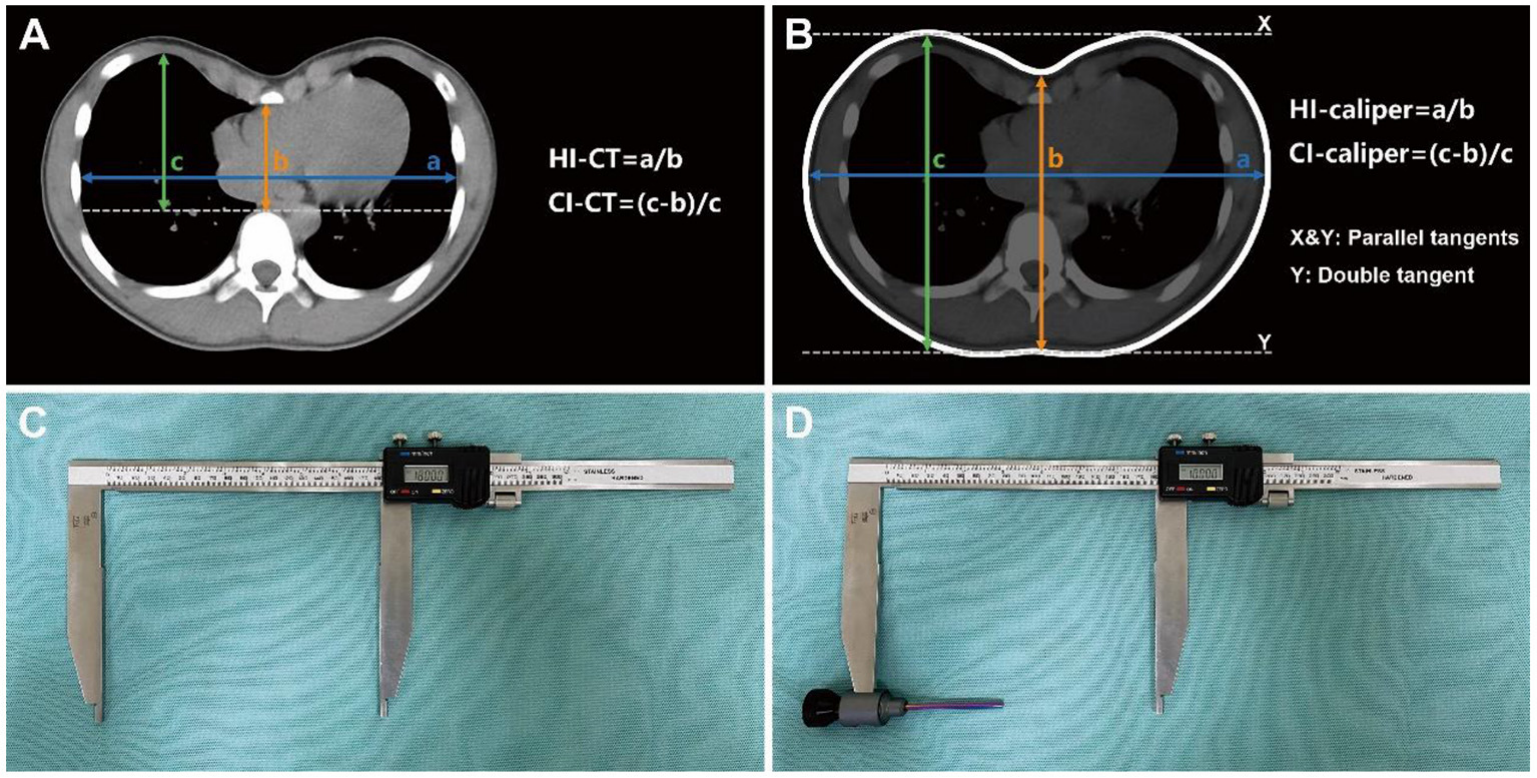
Severity indices and measuring caliper. (A) CT-based severity indices of internal measurement, targeting the bony thorax from pleura to pleura. (B) Caliper-based severity indices of external measurement, targeting the body surface from skin to skin. (C) Caliper for measuring the maximum distance between the parallel tangents of bilateral chest wall (a in B) and the maximum distance between the parallel tangents of anterior-posterior chest wall (c in B). (D) Caliper with “probe” installed for measuring the minimum distance from the deepest point of anterior chest wall depression to the double tangent of bilateral posterior chest wall (b in B).
